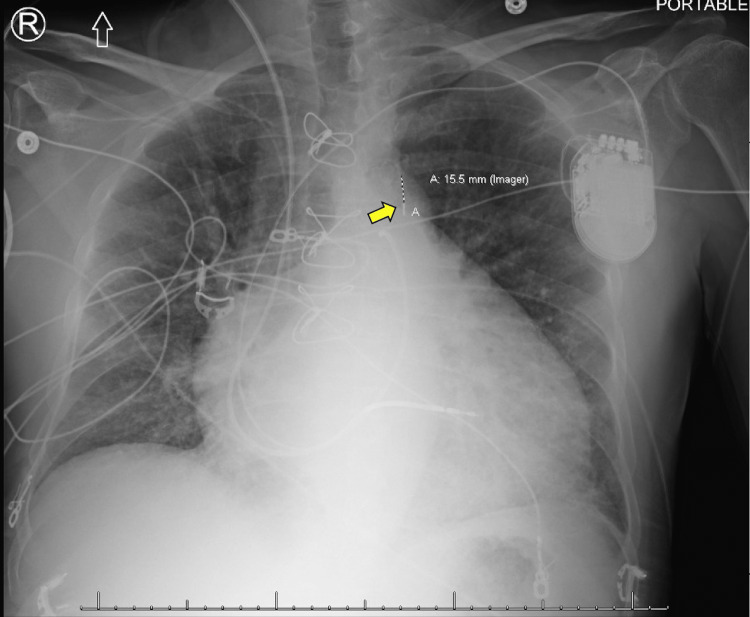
Chest X-ray (anteroposterior view) demonstrating the intra-aortic balloon pump catheter tip (yellow arrow) positioned approximately 1.6 cm from the aortic knuckle.
